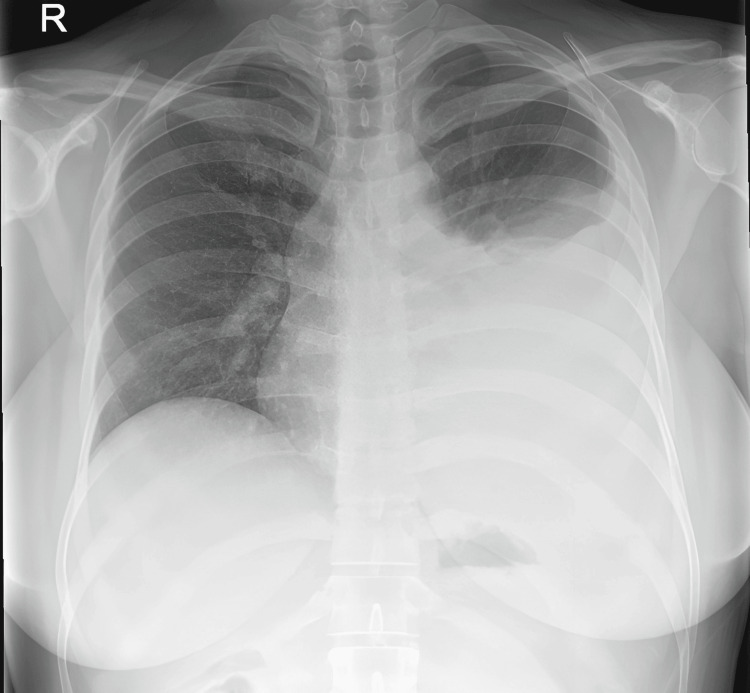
Initial chest radiograph (CXR) demonstrating a left-sided pleural effusion.
